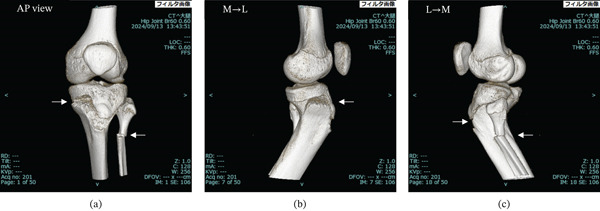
Initial plain CT images of the left knee. (a) Plain CT image of the left knee joint at the initial visit to our department. There is a Salter–Harris Type II fracture (arrow) of the left proximal tibial epiphyseal line and a fracture (arrow) of the proximal fibular shaft. (b) Plain CT image of the left knee joint at the initial visit to our department, medial to lateral view. There is a Salter–Harris Type II fracture (arrow) of the left proximal tibial epiphyseal line. (c) Plain CT image of the left knee joint at the initial visit to our department, lateral to medial view. There is a Salter–Harris Type II fracture (arrow) of the left proximal tibial epiphyseal line and a fracture (arrow) of the proximal fibular shaft.
